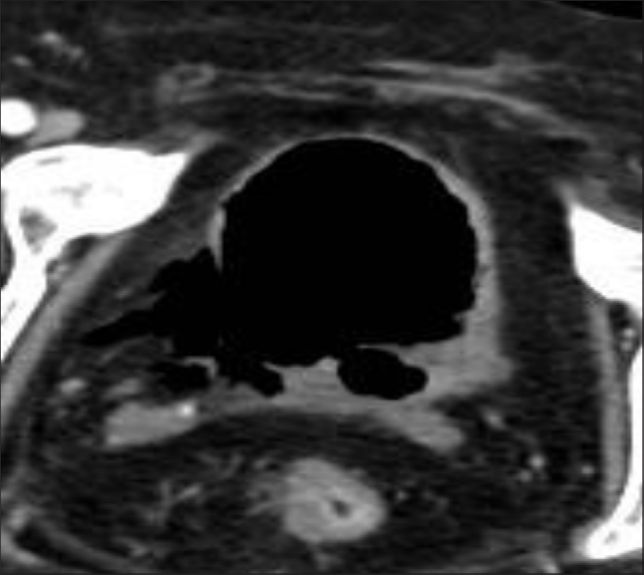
Axial unenhanced CT scan of the abdomen shows a disruption of the bladder wall at the right ­posterolateral side.
